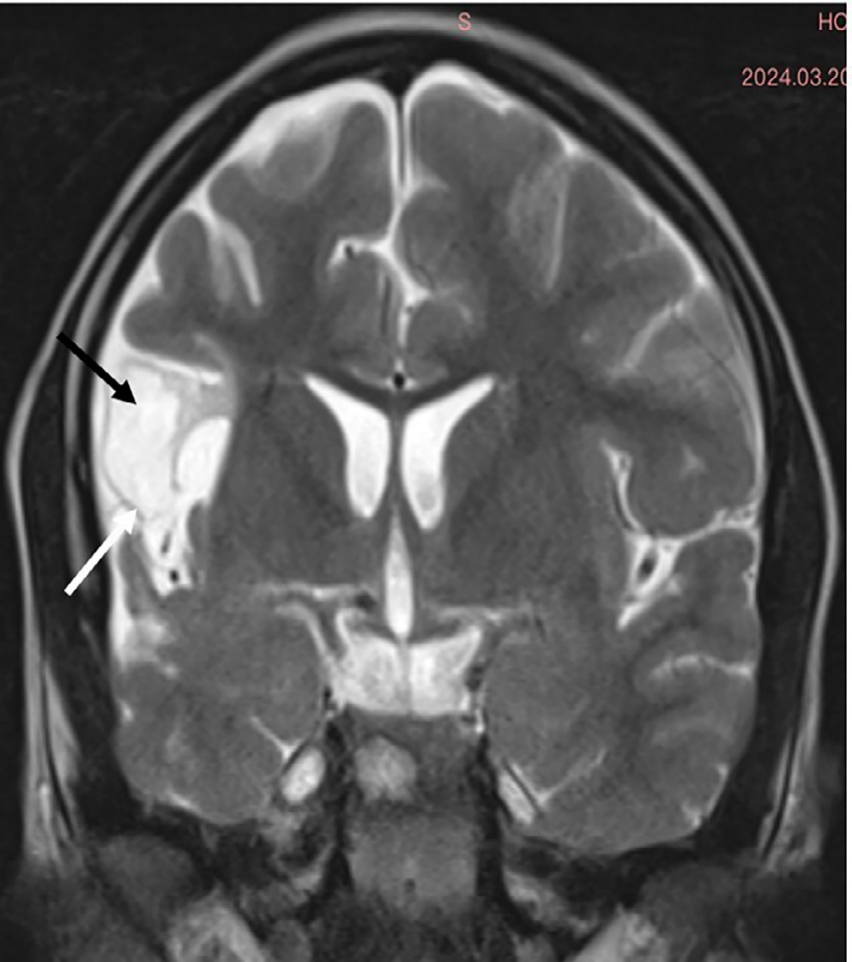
Coronal FLAIR MRI in Sturge-Weber syndrome type III. The image demonstrates hemiatrophy of the right cerebral hemisphere with prominent involvement of temporal and parietal lobes (black arrow), associated with congested veins (white arrow). These findings reflect chronic hypoperfusion and venous congestion typical of SWS.
